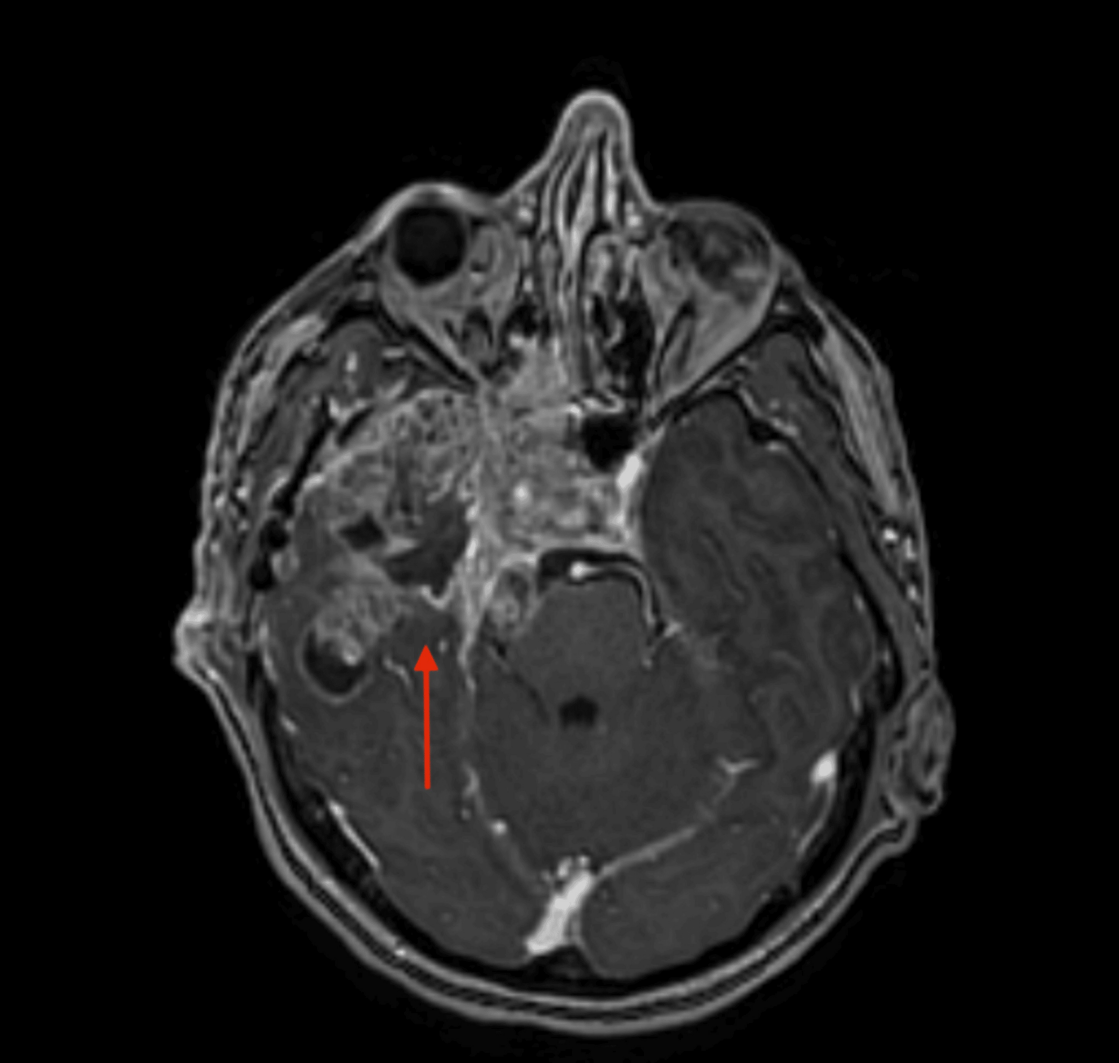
MRI of the brain MRI of the brain shows a large infiltrative tumor in the right temporal lobe extending into the right sphenoid sinus and right retro-orbital region, measuring approximately 6 x 8 cm.
